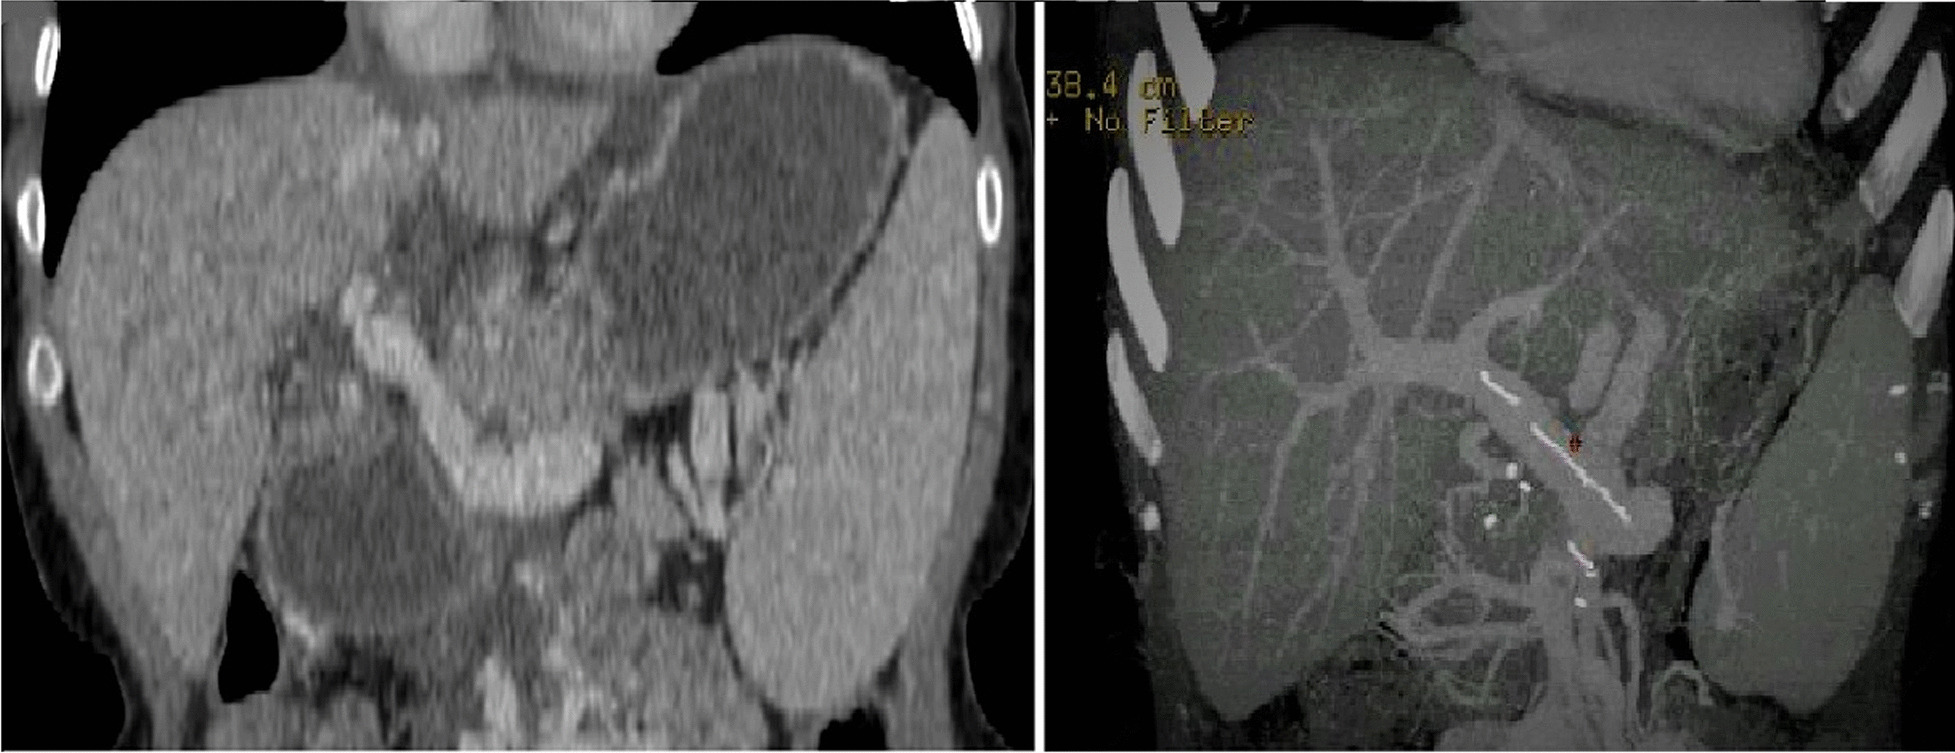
Coronal-MPR and axial-MIP images showing portal vein reconstruction with jumping graft interposition. A, B CT scan MPR images showing portal vein reconstruction with the jumping graft from the superior mesenteric vein
